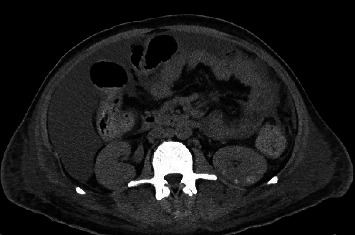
CT of the abdomen showing diffuse irregular and nodular gastric wall thickening.
